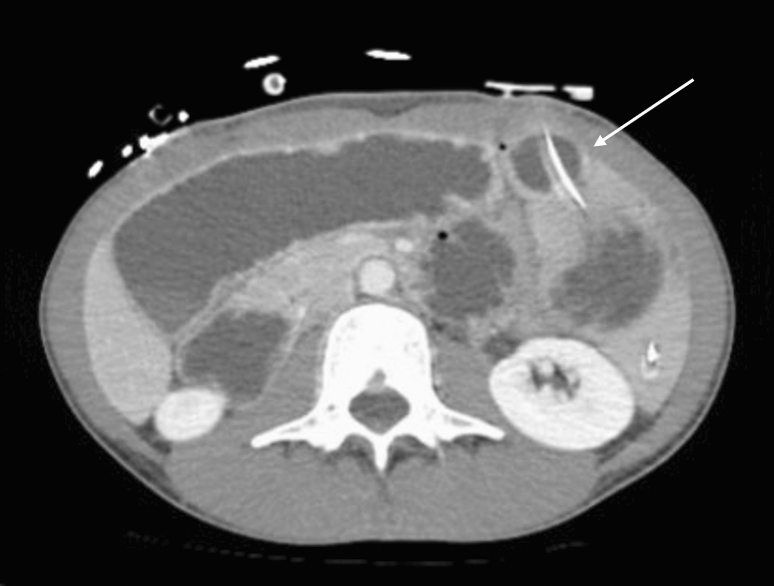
Axial computed tomography demonstrating dilation of the proximal jejunum and duodenum adjacent to the jejunostomy tube balloon (arrow).
